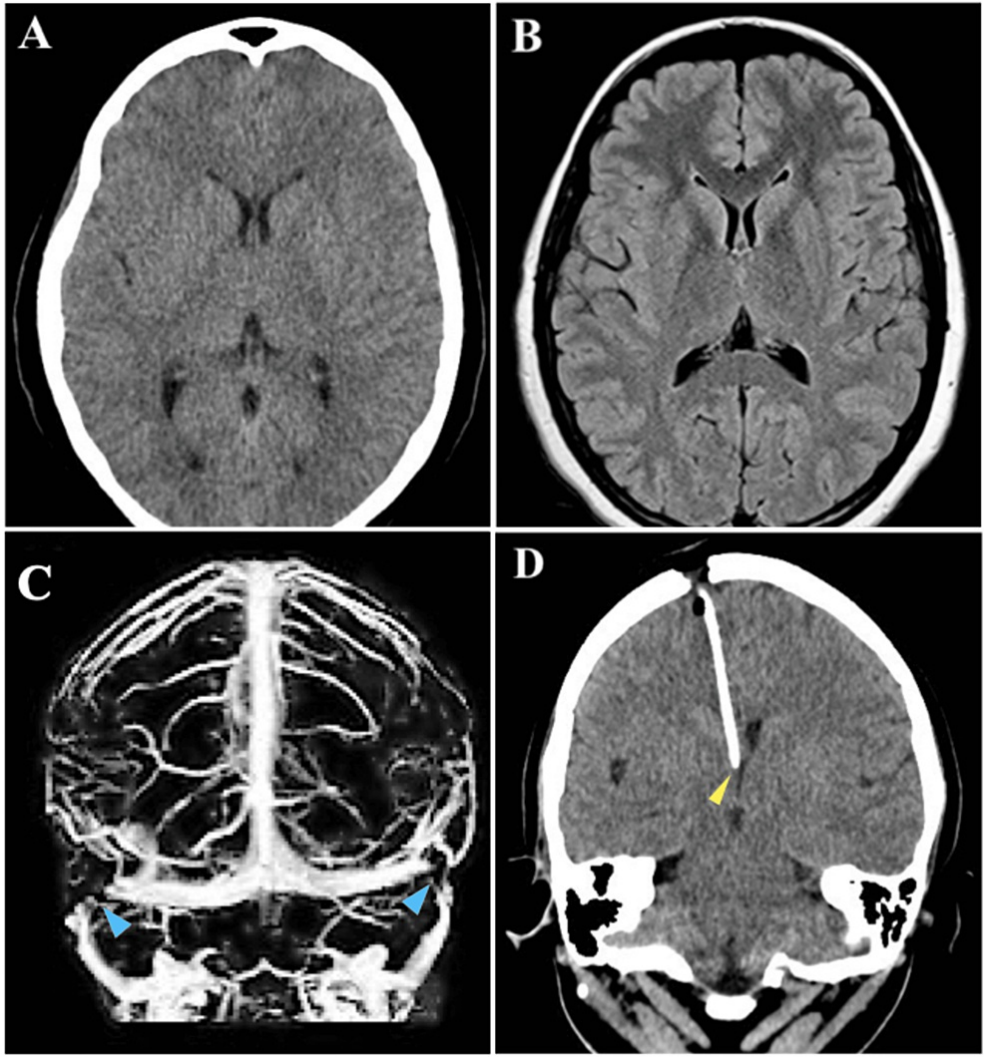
Case 2 Axial brain CT scan (A) and MRI (B) show a slit-like ventricle pattern. (C) Brain MRI venous angiography shows bilateral sinus stenosis between the transverse and sigmoid sinuses (blue arrowheads). (D) Postoperative coronal CT scan shows accurate ventricular catheter position in a sufficient pool of CSF with the tip in the foramen of Monro (yellow arrowhead).
